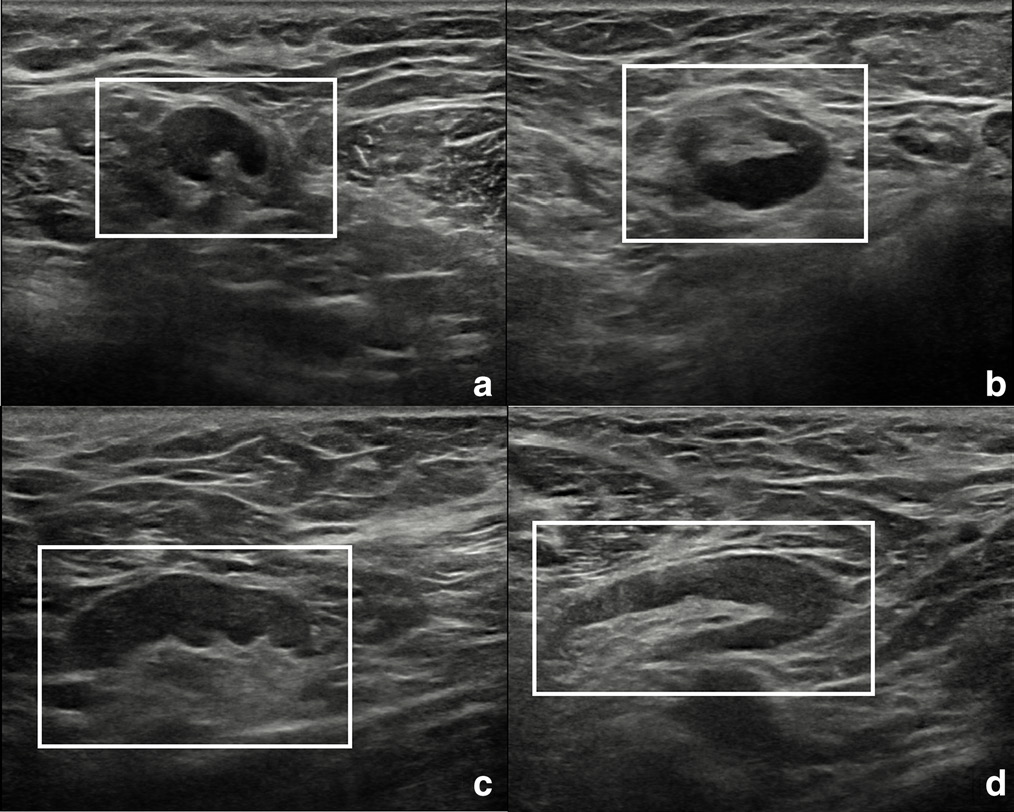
Ultrasound scans of right (A, B) and left (C, D) armpits showing lymph nodes with short to long axes ratio <0.5 (white rectangles). Oval shape, bilateralism, and visible fatty hilum are suggestive for a benign, reactive aetiology.
